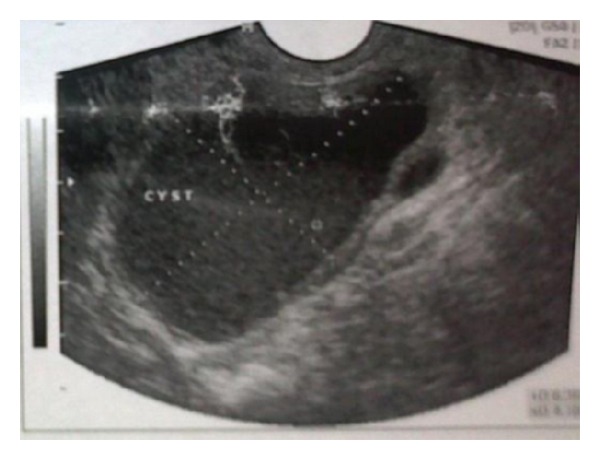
Transrectal ultrasonography of the left seminal vesicle cyst.
